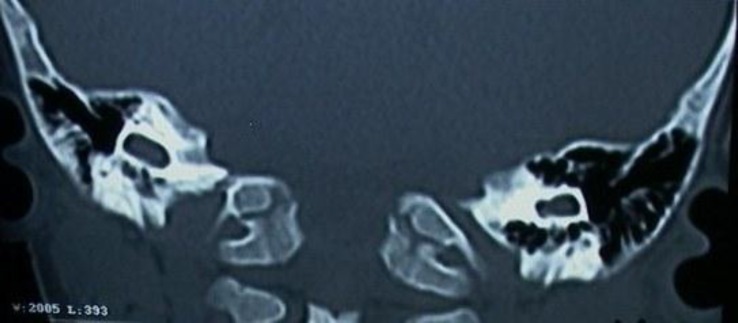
HRCT temporal bone showing an irregular single cavity on the lateral aspect of internal auditory canal bilaterally. No cochlear turns could be identified
